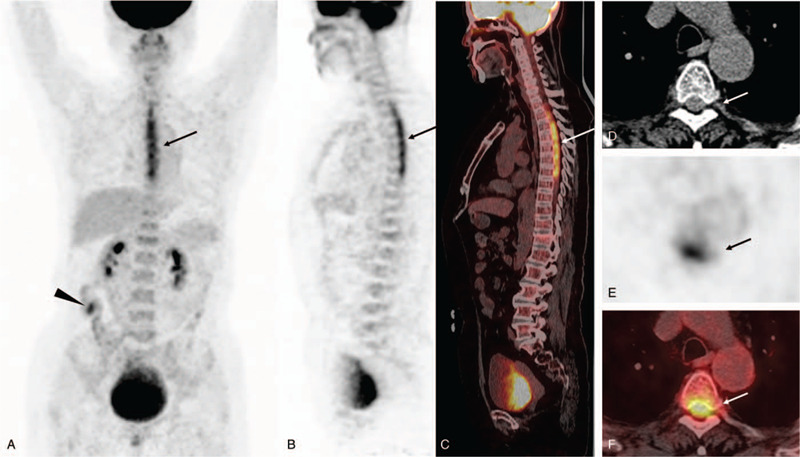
^18^F-FDG PET/CT findings. (A) MIP of FDG-PET showed intense hypermetabolism in the spinal cord (arrow) and an FDG-avid focus in the ascending colon (arrowhead); (B, C) Sagittal PET and fusion images showed increased FDG uptake in the spinal cord between the level of 1st and 7th thoracic vertebra (SUVmax 5.4, arrows); (D-F) Axial CT, PET and fusion images at the level of T4 showed diffuse intraspinal hypermetabolism (arrows).
